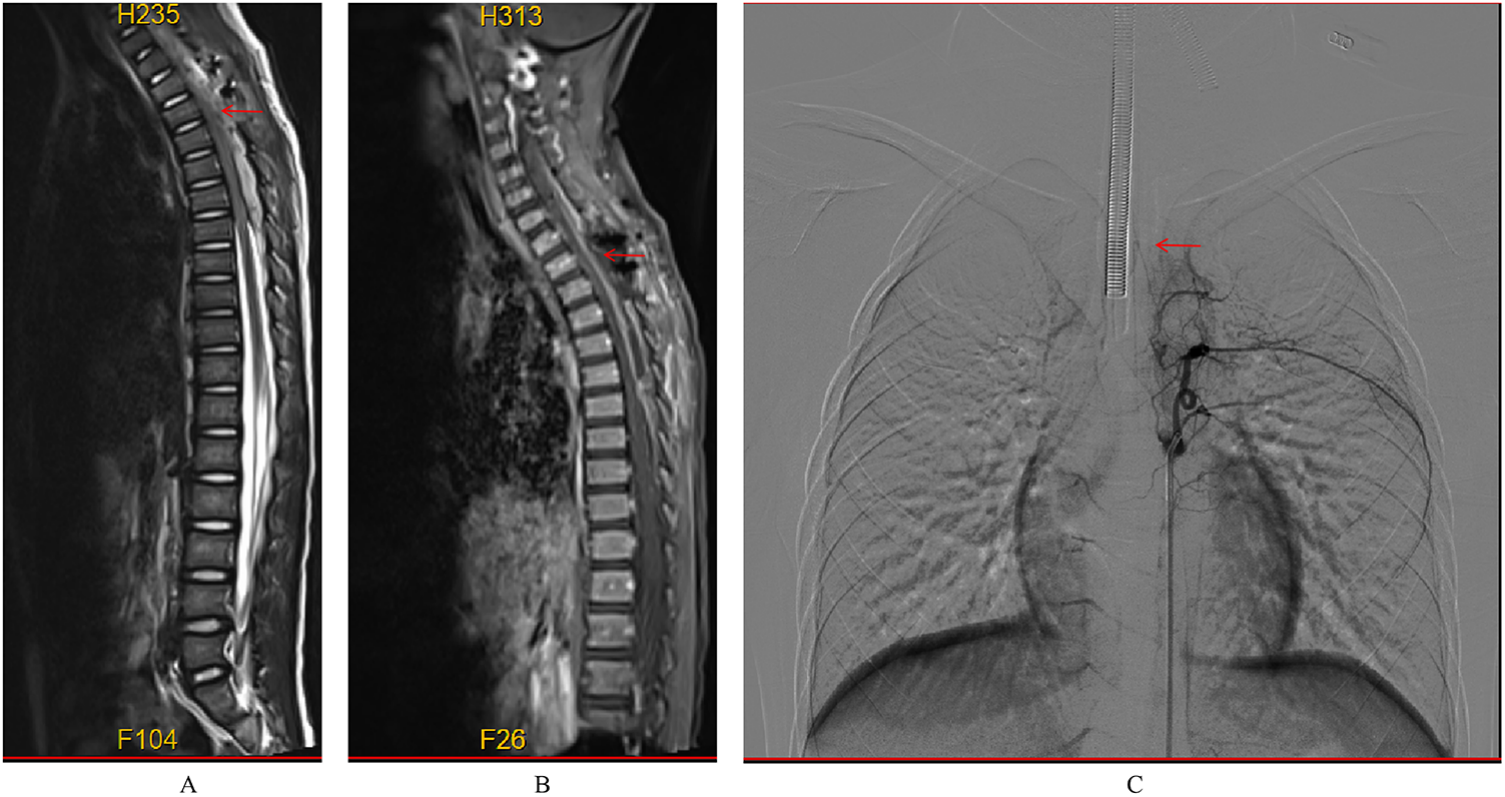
Postoperative MRI did not reveal any significant abnormal signals (A,B); postoperative DSA showed no evidence of abnormal vasculature (C).
